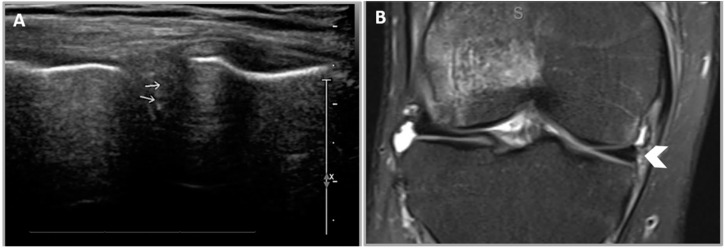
False-positive medial meniscal tear of the right knee in US. (A) US image of the right medial meniscus demonstrating small meniscal clefts (white arrows). (B) A coronal proton density MRI image demonstrating a normal medial meniscus (white chevron).
